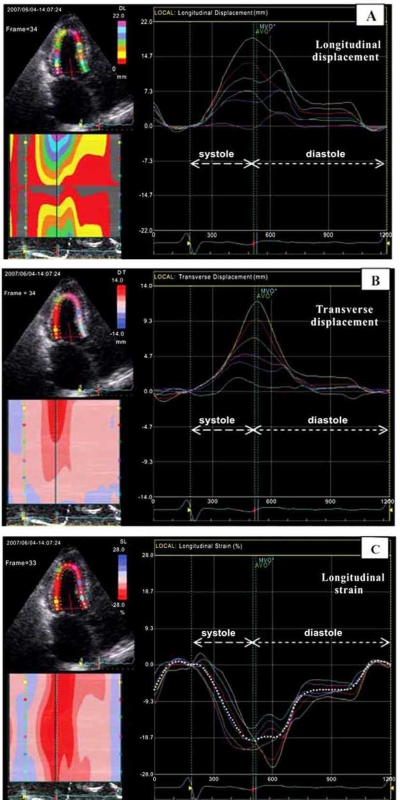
Speckle-tracking 2D-strain imaging (apical long axis view) in a heart transplanted patient with normal LV function and no angiographic evidence of coronary artery disease. The same echocardiographic loop was used for evaluation of myocardial displacement and longitudinal deformation (strain) in the 6 visible LV wall segments. The images in panel A and B show that myocardial displacement can be misleading by suggesting regional differences in contractile function, although, as shown in panel C, myocardial deformation analysis (strain imaging) does not reveal relevant regional differences in myocardial longitudinal shortening.
